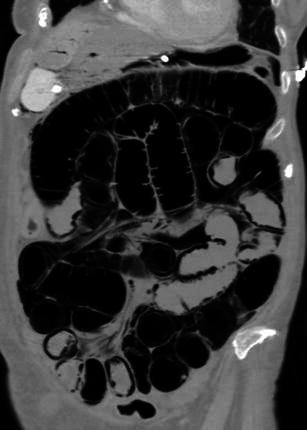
Dilated bowel in acute ischaemia. Coronal reformatted CT image demonstrates diffuse small bowel dilation with pneumatosis in a patient with extensive small bowel ischaemia. Portal venous gas in the liver is also evident
